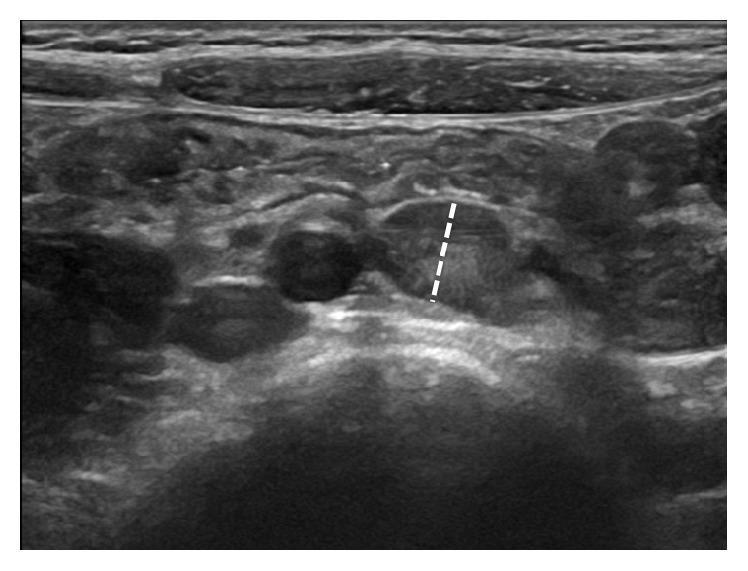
Abdominal ultrasound showing large hypoechoic mesenteric lymph nodes in a 6-year-old girl with acute nonspecific mesenteric lymphadenitis. The largest mesenteric lymph node short-axis diameter (dashed line) measurement was 9 mm.
